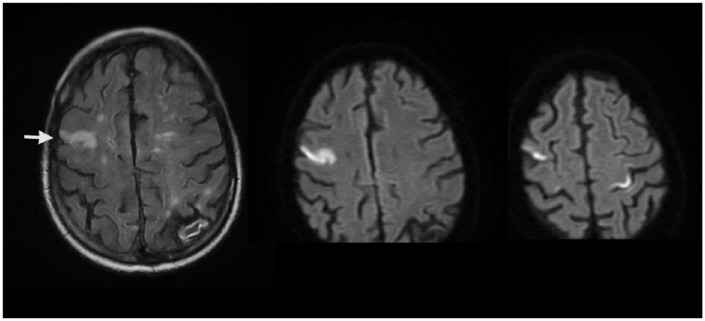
Magnetic resonance imaging demonstrating acute ischemic stroke. Left, T2 FLAIR image showing a high signal lesion in the right frontal lobe. Center and right, diffusion-weighted images showing bilateral positive gyriform signals in the right and left posterior frontal lobes.
